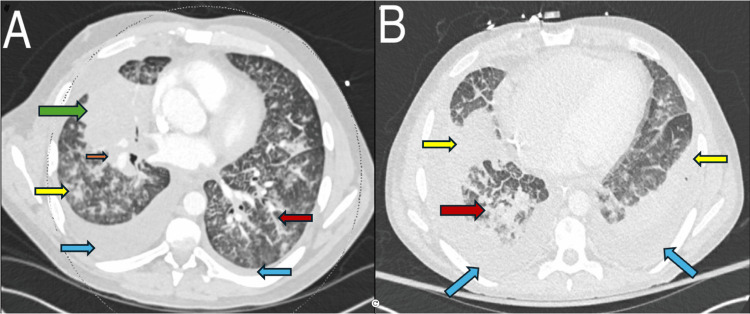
Contrast-enhanced CT of the chest (A) and and abdomen/pelvis (B) A: Contrast-enhanced CT chest showing right lung consolidation (green arrow) with nodular opacities (yellow arrow) and interlobular septal thickening (red arrow), mediastinal lymphadenopathy (orange arrow), and pleural thickening; B: Repeat contrast-enhanced CT scan chest/abdomen/pelvis showing worsening multifocal airspace disease (red arrow) (thickened interlobular septa with ground-glass opacities), bilateral consolidations (yellow arrows), and pleural thickening with new bilateral pleural effusions (blue arrows)
